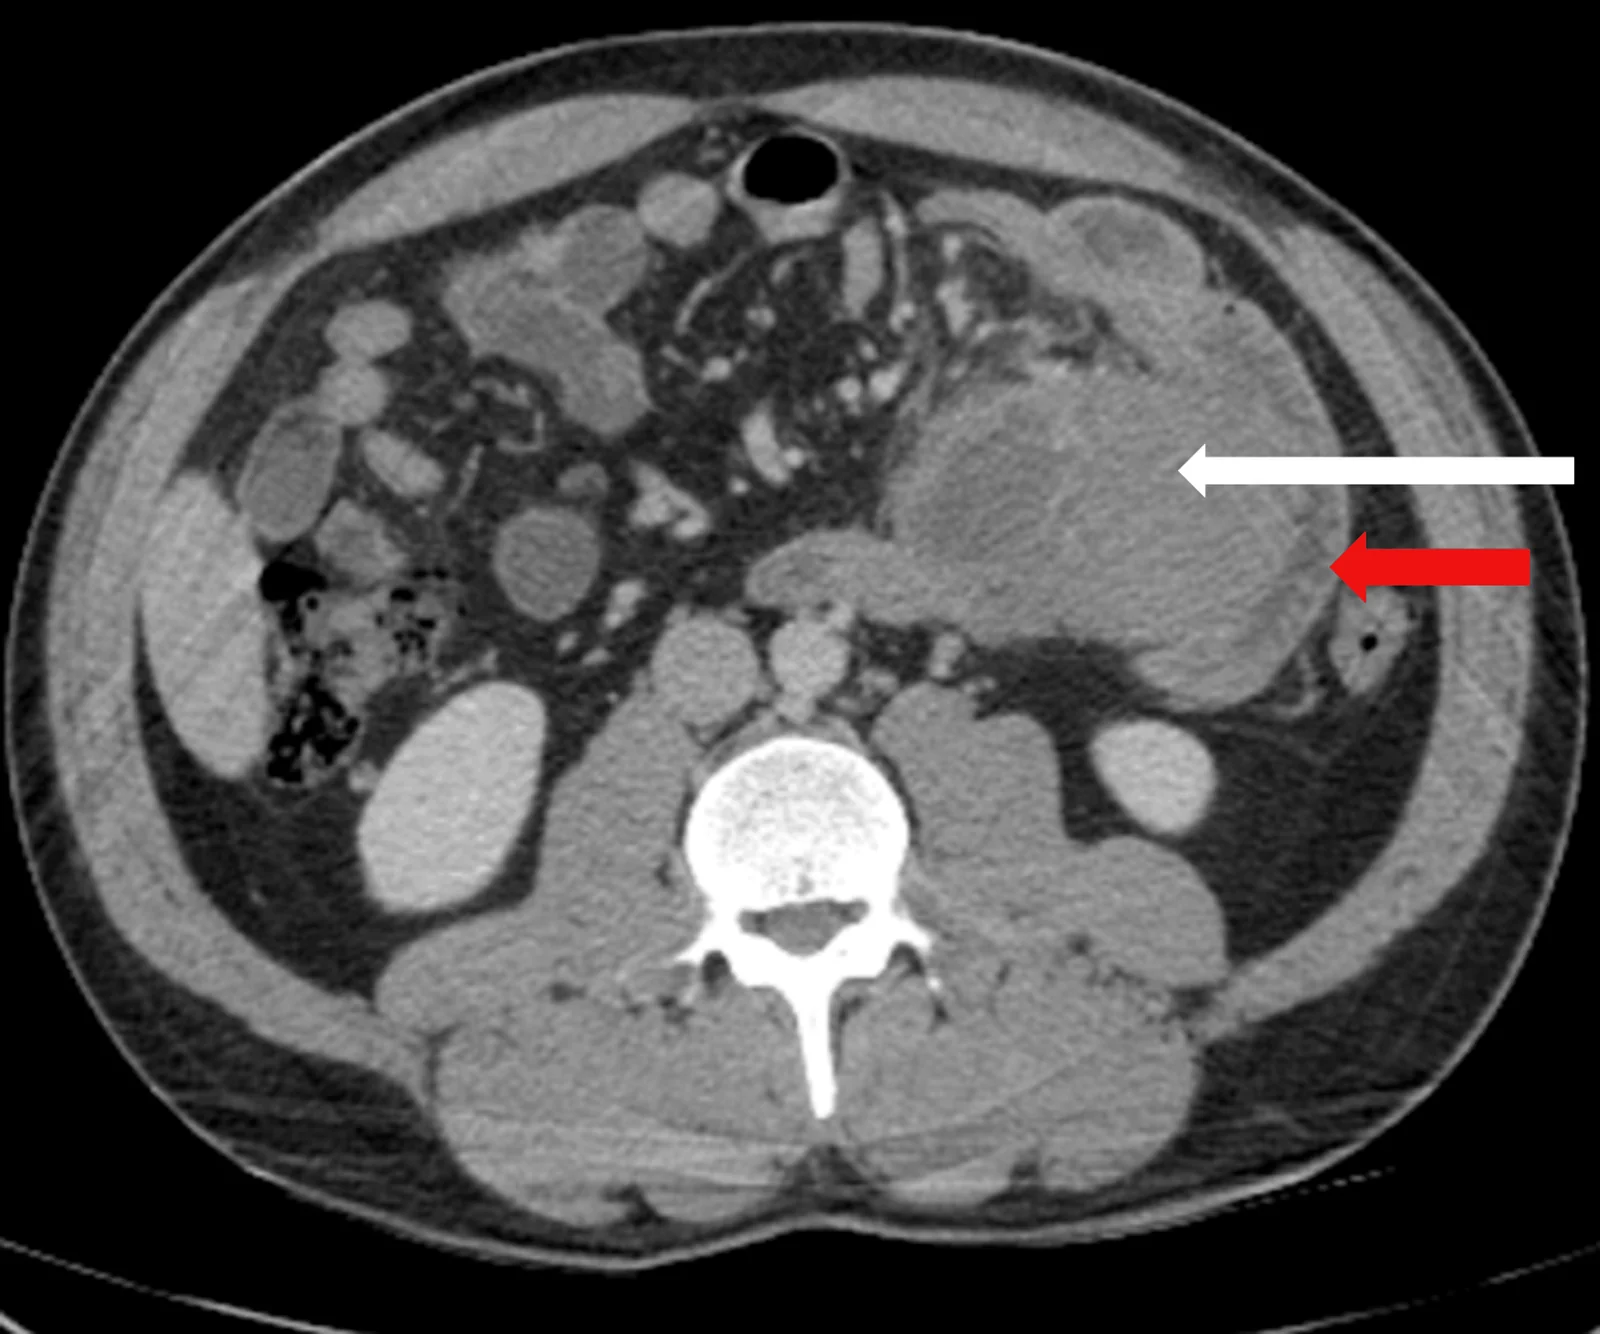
Axial CT image demonstrating a heterogeneous mass (white arrow) within the mesentery, displacing adjacent jejunal loops (red arrow)
